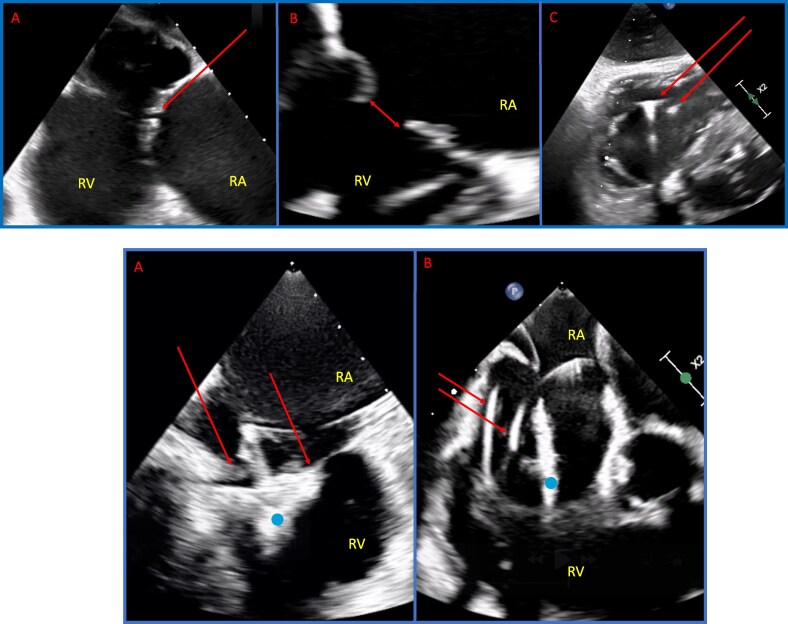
Anatomical and imaging considerations for transcatheter tricuspid valve interventions. Upper left: Transesophageal images showing adequate image quality with desirable anatomical features for T-TEER with good leaflet length, mobile leaflets, and a reasonable coaptation gap (red arrow); Upper middle: Transesophageal echocardiographic images showing sub-optimal anatomical features for tricuspid TTEER, with a wide coaptation gap (red arrow). This patient was referred for TTVR; Upper right: Transesophageal echocardiographic images showing sub-optimal image caused by artefact from two transvalvular pacing leads (red arrows). This patient underwent T-TEER using ICE, see lower panel. ICE images demonstratings the advantage of intracardiac imaging in challenging anatomies. Lower left: Clear images of the clip (blue dot) and valve leaflets (red arrows) during T-TEER, despite the presence of pacing leads, in the patient from Figure 1C. Lower right: Images of pacing leads (red arrows) and clip/delivery (blue dot) catheter in the same patient. ICE, intracardiac echocardiography; RA, right atrium; RV, right ventricle; T-TEER, transcatheter tricuspid valve edge-to-edge repair; TTVR, transcatheter tricuspid valve replacement; TV, tricuspid valve.
